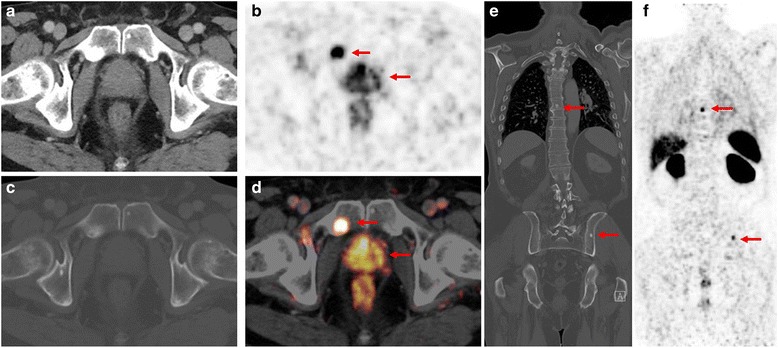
^68^Ga-PSMA ligand PET/CT in a 78-year old patient with primary PC (Gleason score 7, iPSA 34.3 ng/ml). The patient presented with intense, multifocal uptake in the prostate b, d without correlate on CT images a The CT examination with bone window setting c and e shows osteoblastic bone lesions in the left iliac bone as well as in the thoracic vertebrae with corresponding PSMA-ligand uptake in PET imaging f However, the focal PSMA-ligand uptake in the right symphysis (B, D) shows only a slight hypersclerosis (C) on corresponding CT images that might have been missed without PET. Note the physiological high uptake in the kidneys as well as moderate uptake in the liver and spleen (F)Finally, it has to be noted that not all PC exhibit a significant PSMA overexpression. In a study of Maurer et al. about 8 % of patients with primary PC did not show PSMA overexpression—with currently no specific biological explanation [16]. Therefore, correct and careful interpretation of the diagnostic CT scan as part of the ^68^Ga-PSMA ligand PET/CT examination is of special importance.Pitfalls of ^68^Ga-PSMA ligand PETAs ^68^Ga-PSMA ligand PET/CT imaging is a relatively new imaging technique it is important to be aware that ^68^Ga-PSMA ligands are not completely specific for PC to avoid scan misinterpretation. So far, several case reports exist showing increased PSMA uptake in benign lesions such as thyroid adenoma, Paget’s disease, schwannoma, tuberculosis, adrenal adenoma or splenic sarcoidosis [22, 34–36]. Further, it is known that coeliac ganglia show a relevant ^68^Ga-PSMA uptake. In a study of Krohn et al. at least one ganglion with tracer uptake was found in 76/85 patients (89.4 %) undergoing ^68^Ga-PSMA ligand PET/CT examination which may mimic lymph node metastases in this area [37]. An example of a ^68^Ga-PSMA ligand PET/CT examination with focal uptake in the coeliac ganglion is presented in Fig. 4. Interestingly, PSMA expression has also been reported in the tumor neovasculature of some solid tumors (e.g. colon, breast, renal) and in newly formed blood vessels. Apart from PC, other malignant lesions presenting with increased PSMA expression have been reported for glioblastoma, hepatocellular carcinoma, lung cancer, renal cell carcinoma and thyroid cancer [38–42]. For example, differentiation between lung metastases and primary lung cancer which can also occur in elderly patients as secondary malignancy is a common clinical question. A study by Pyka et al. revealed that quantitative (SUV) analysis of ^68^Ga-PSMA ligand PET was not able to discriminate reliably between pulmonary metastases and primary lung cancer in PC patients as primary lung cancer lesions can also show high PSMA-expression by ^68^Ga-PSMA ligand PET. [41]. In summary, in unclear ^68^Ga-PSMA ligand PET positive lesions, morphological correlation, further clarification with other imaging techniques such as MRI, ultrasound or biopsy is mandatory.
